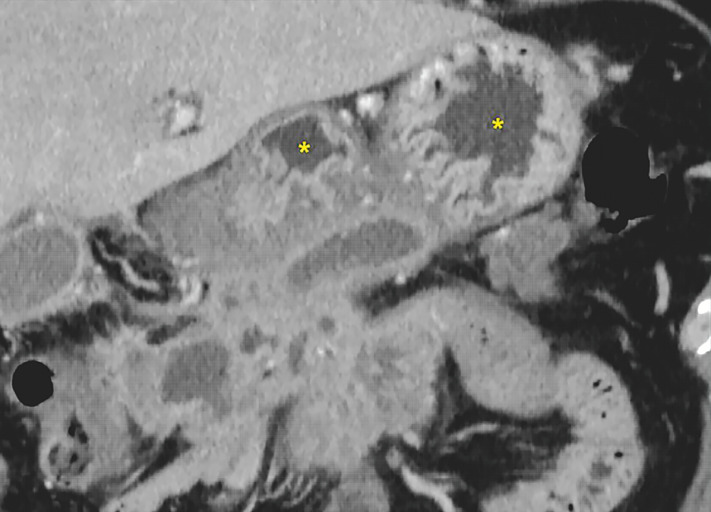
Computed tomography delineating a large walled-off necrotic lesion with a maximum diameter of 13 cm and edematous thickening of the gastric wall (asterisks).
